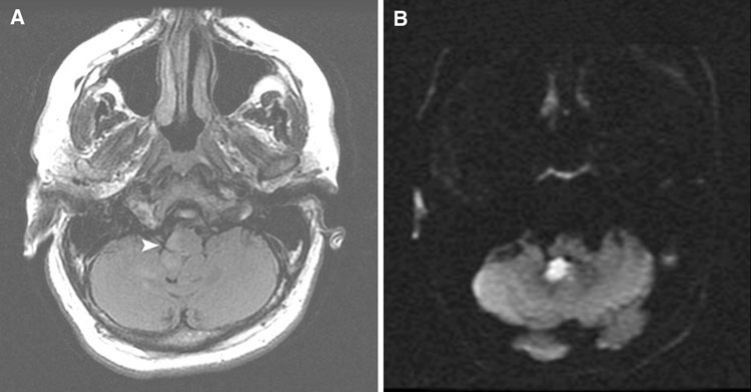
Magnetic resonance imaging (MRI). a Axial T2-weighted FLAIR MRI shows slight hyperintensities in the right lateral medulla and right cerebellum. b Diffusion-weighted MRI demonstrates restricted water motion in the lesion shown in a, indicating recent infarction
